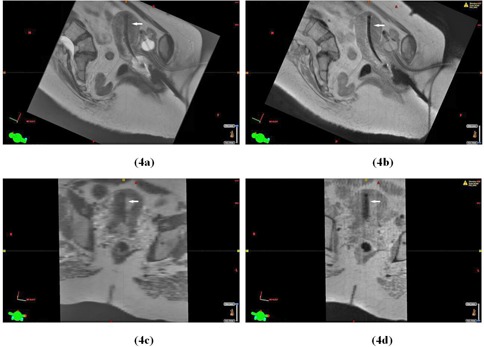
A parasagittal slice displaying the tandem (as indicated by the arrow) relative to the surrounding anatomy in the T2W‐MRI (a) and in the corresponding slice in the PDW‐MRI (b) for Patient 3. For the same dataset, a paracoronal slice displaying the tandem (as indicated by the arrow) in the T2W‐MRI (c) and in the corresponding slice in the PDW‐MRI (d).
